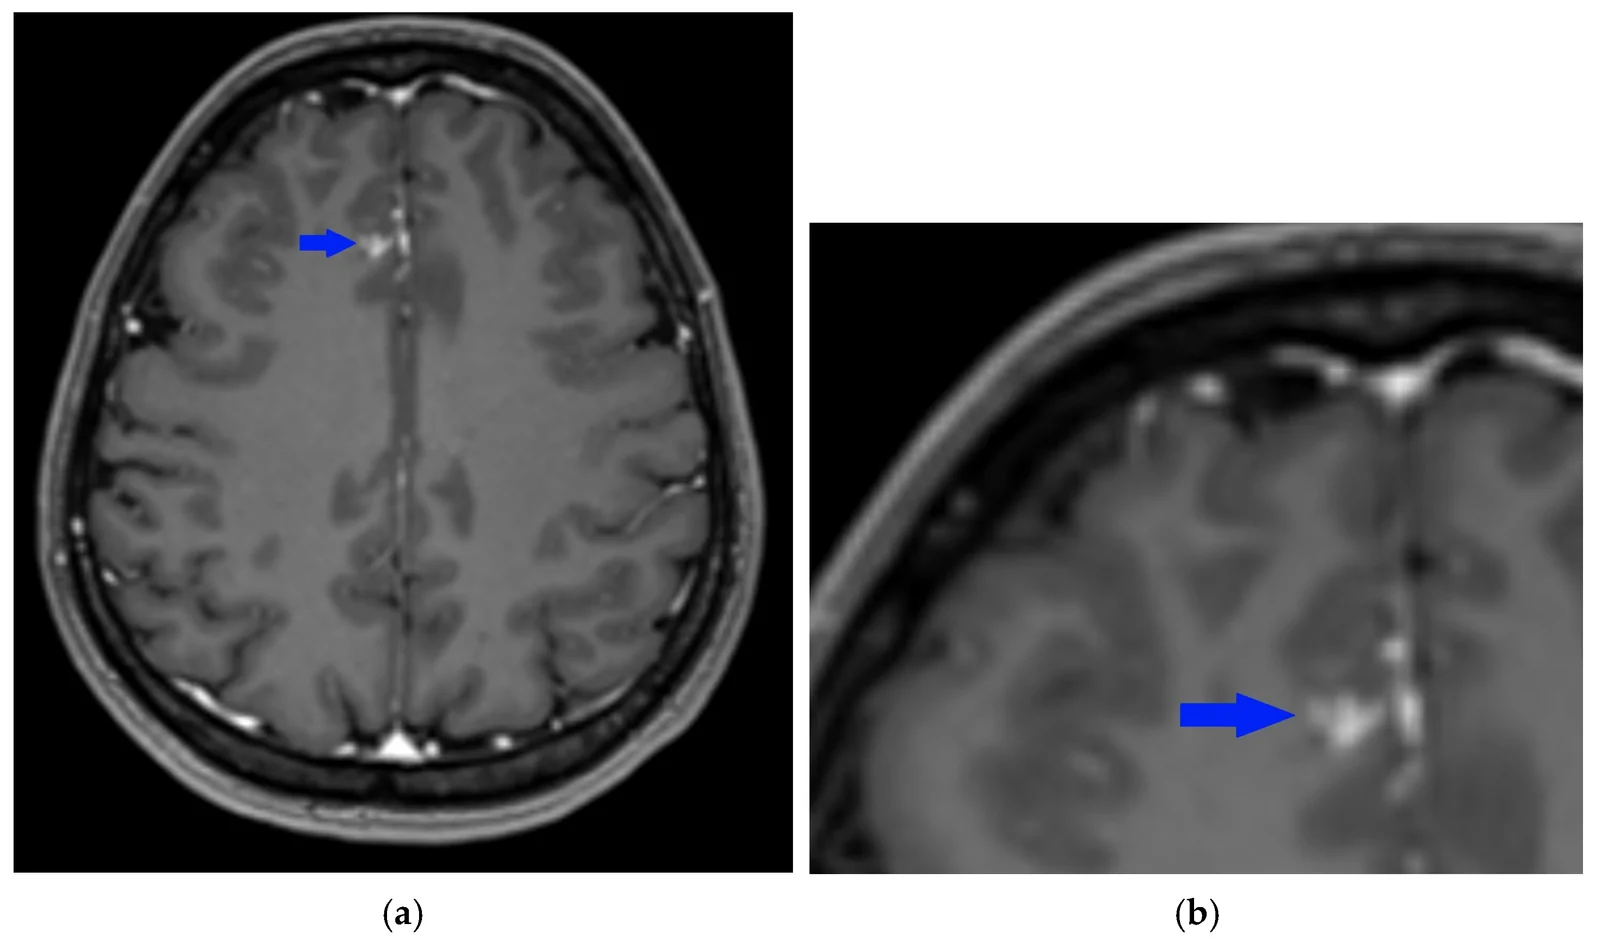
A 40-year-old woman was seen in the ophthalmology clinic complaining of intermittent visual disturbances and a headache. Brain MRI revealed a small, round area of contrast enhancement suggesting a vascular malformation—marked with blue arrow (a,b). At that time, she had one episode of intermenstrual bleeding. Apart from that, the patient had regular 28-day menstrual cycles. She had a history of one uncomplicated pregnancy and one full-term vaginal delivery five years ago.
